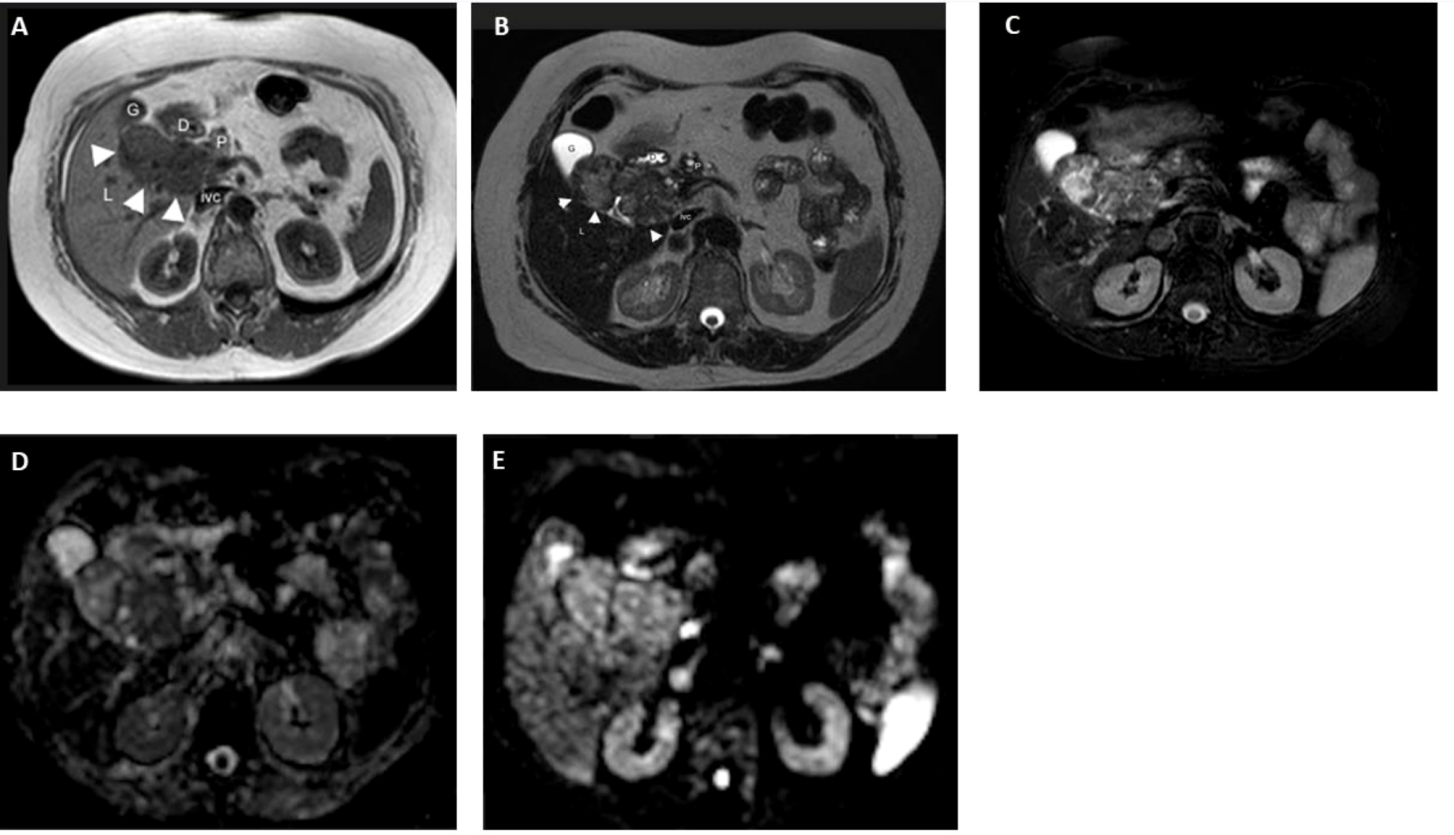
MR in a 65-year-old woman with ectopic thyroid in the hepatic hilum. (A) Axial T1 image. Dishomogeneously hypointense (with respect to the liver) mass in the right hypochondrium between the duodenum (D), liver (L), pancreas (P), gallbladder (G), and inferior vena cava (ivc). Axial (B) T2 and (C) T2 fat-sat images. The mass is dishomogenously hyperintense with respect to the liver. (D) DWI B800 image. The mass shows no meaningful restriction. (E) ADC image. High ADC in the mass. (F) MR-Cholangiography. Common bile duct (cbd) compressed and dislocated by the bilobated mass (m); C, choledocus; G, gallbladder; M (mass); Wirsung (arrowheads).
